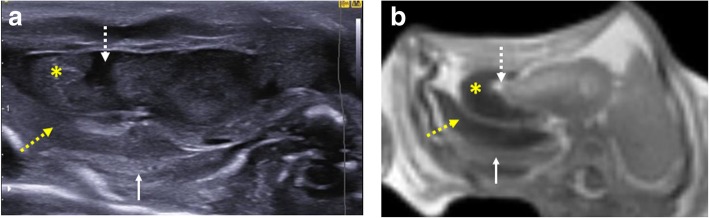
Transverse imaging through the lower thoracic cavity in a 20-week gestational-aged foetus, 2 days after death following termination of pregnancy for suspected renal agenesis. Termination was performed by in utero foetal intra-cardiac injection of potassium chloride. Postmortem ultrasound (a) and postmortem T2-weighted MRI at 1.5 T (b) both demonstrate haemorrhage within the right pericardial (yellow asterisk) and pleural space (yellow dotted arrow). It is also possible to visualise the ‘normal’ consolidated right lower lobe of the lung (white solid arrow) and a small pericardial effusion (white dotted arrow). These are typical foeticide-related changes and iatrogenic in nature
